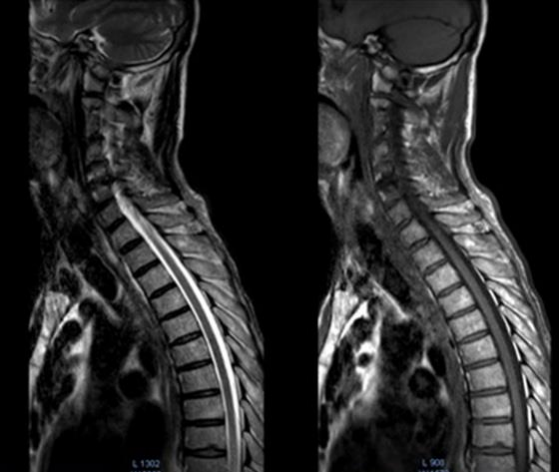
T1 and T2 weighted magnetic resonance imaging; images show a normal thoracic marrow
